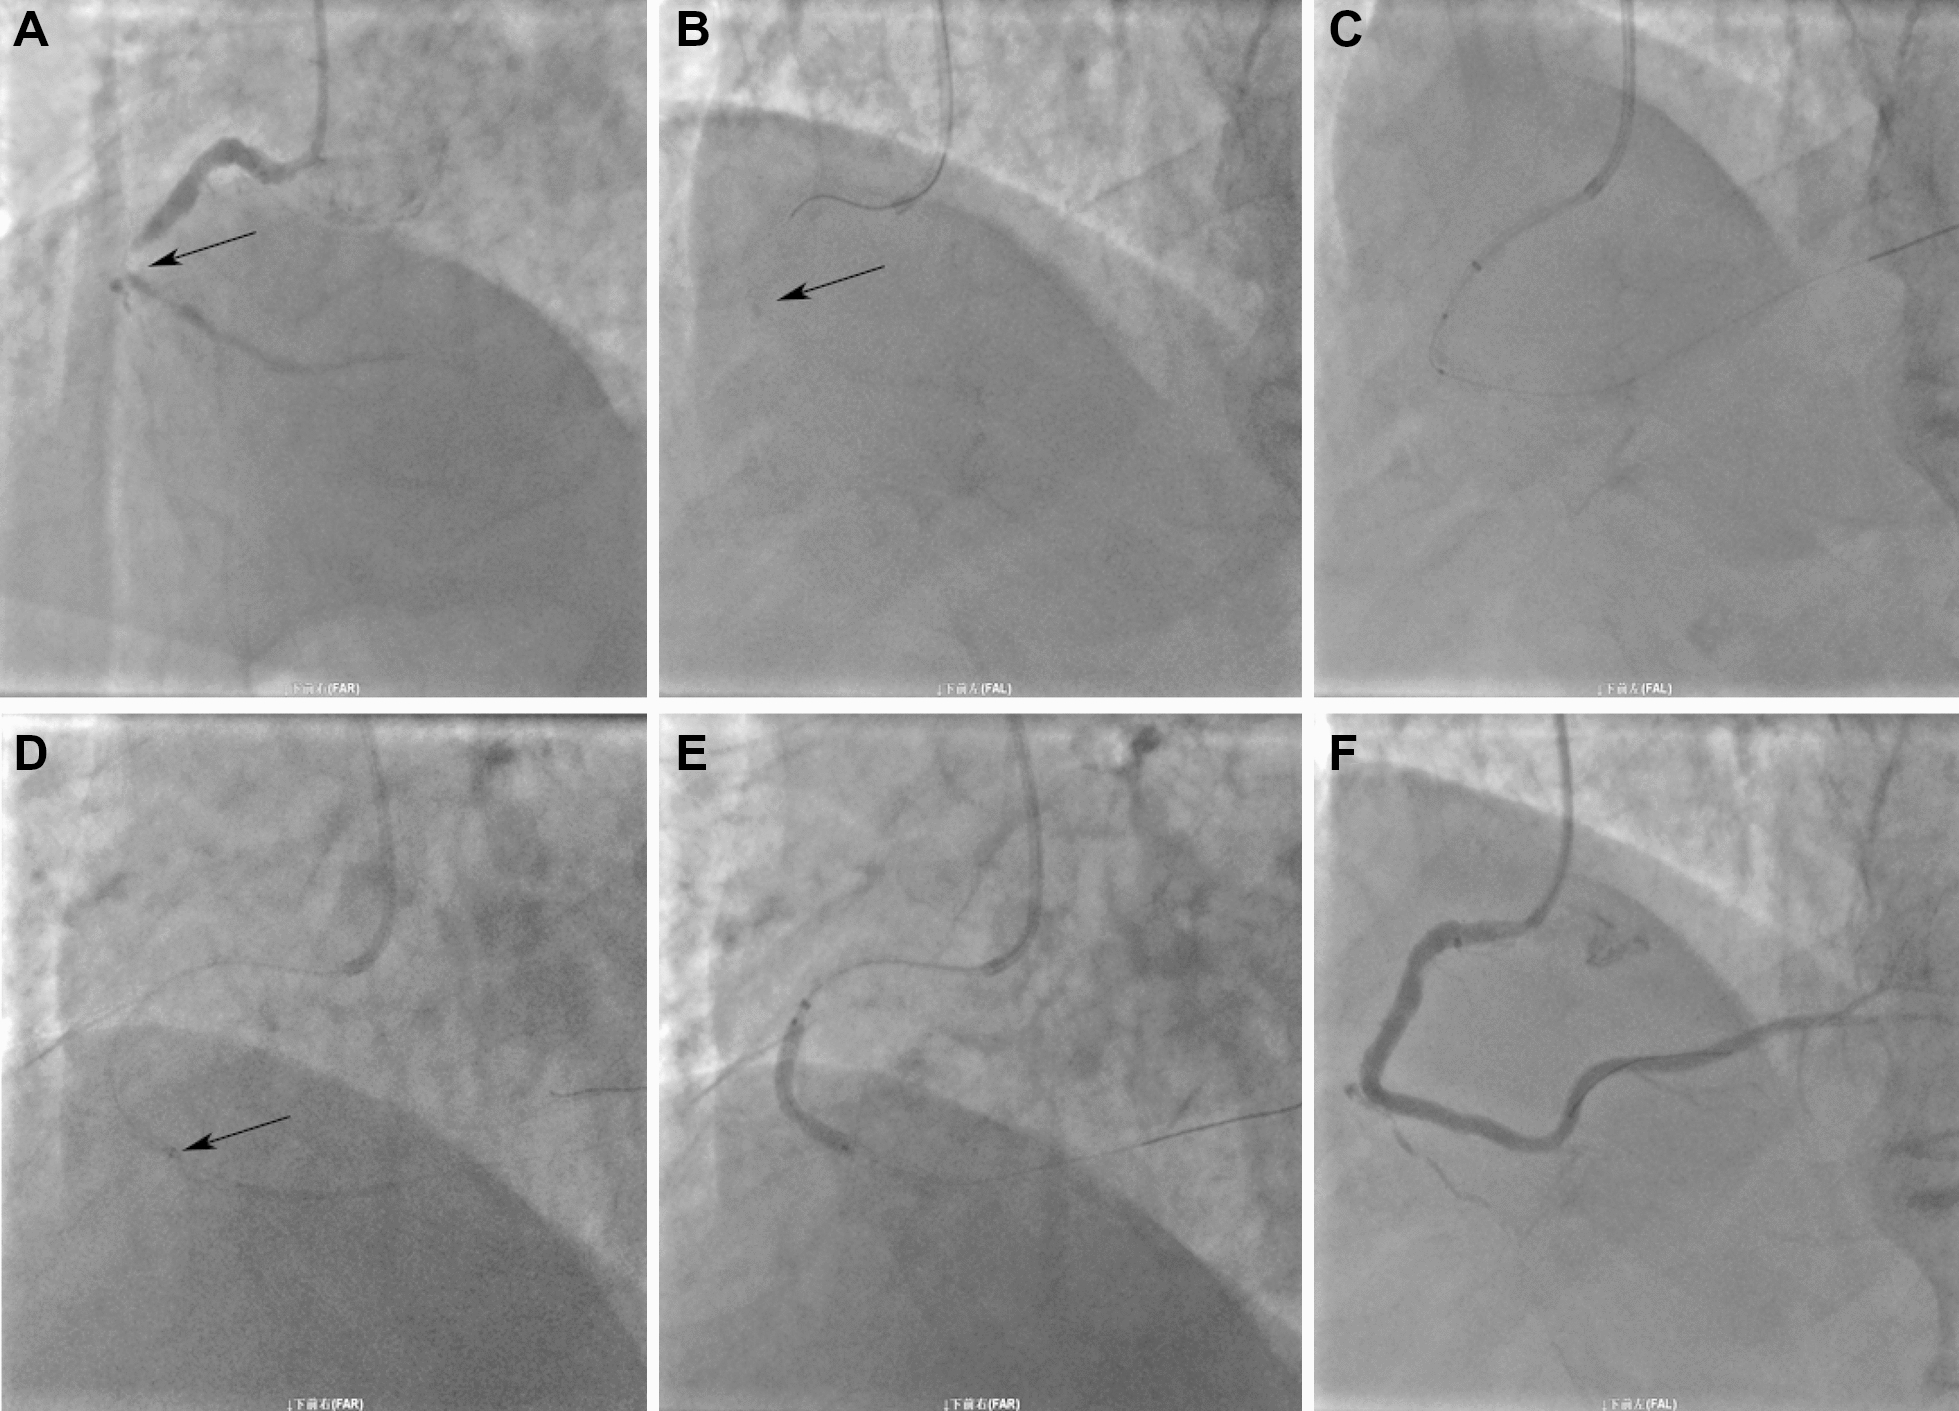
The use of 5-4F tapered Expressman GEC for severely calcified lesion. A Angiography indicated a subtotal occlusion in the right coronary artery (arrow). B X-ray showed calcified lesion with angulation. C With the support of 5F Guidezilla GEC, the lesion was dilated using a 2.0 mm and 2.5 mm balloon. However, the Resolute stent (3.0 × 22 mm) failed to cross the lesion. The 5F GEC was hampered at position of calcified lesion. D The 5-4F tapered GEC was advanced beyond the lesion with an anchor balloon technique. The stent was delivered beyond the target lesion via GEC. The arrow indicates the tip of the GEC. E The Resolute stents (3.0 × 22 mm and 3.5 × 22 mm) were positioned and deployed in the target lesion. F The final angiogram showed successful deployment of the 2 coronary stents with no visible arterial dissection
